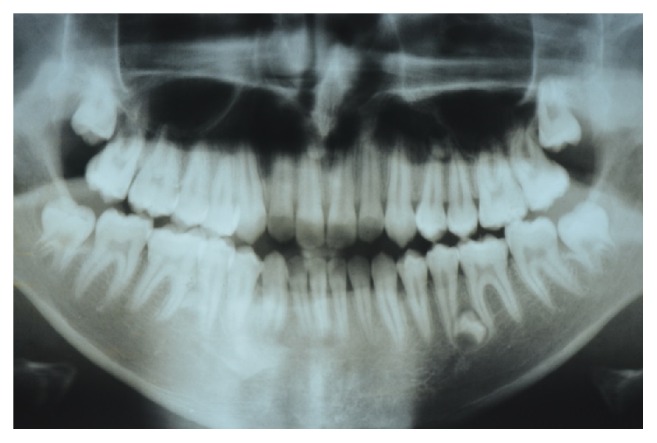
Panoramic radiograph of the patient aged 16 years showing the early formation of 3 supernumerary teeth in the right and left mandibular premolar region and in the left maxillary premolar region.
